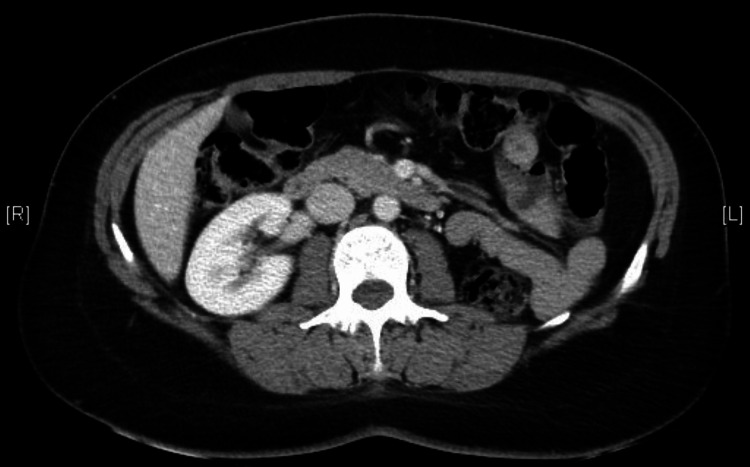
Follow-up computed tomography scan
